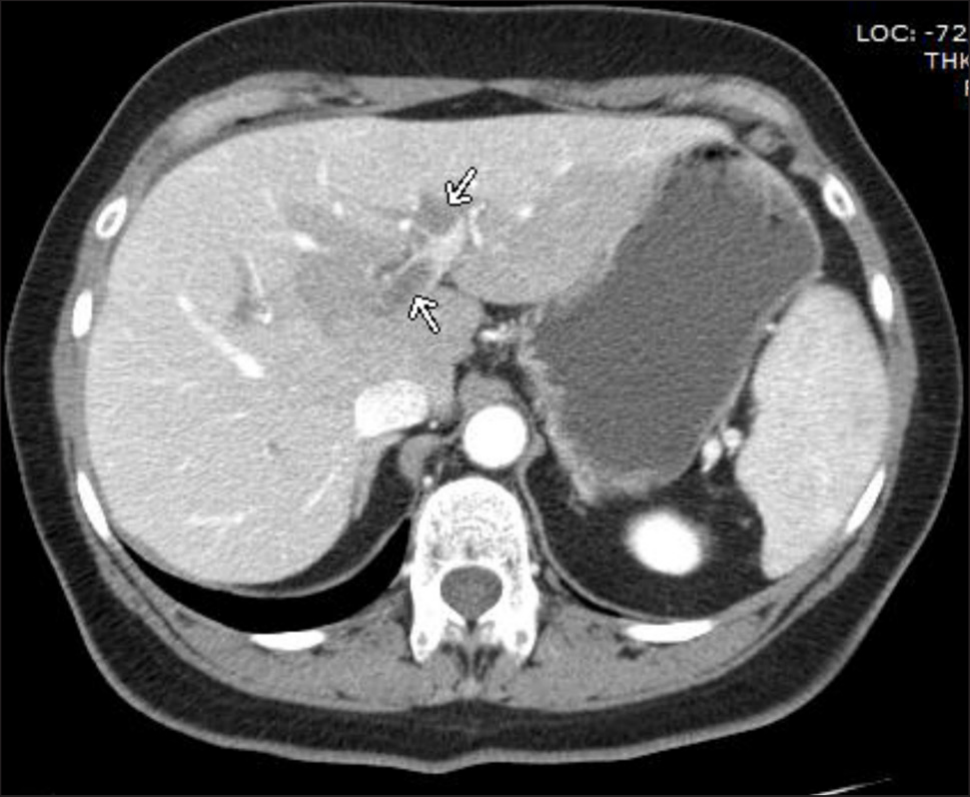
Axial CT image showing the thrombi within the portal veins (arrows).
